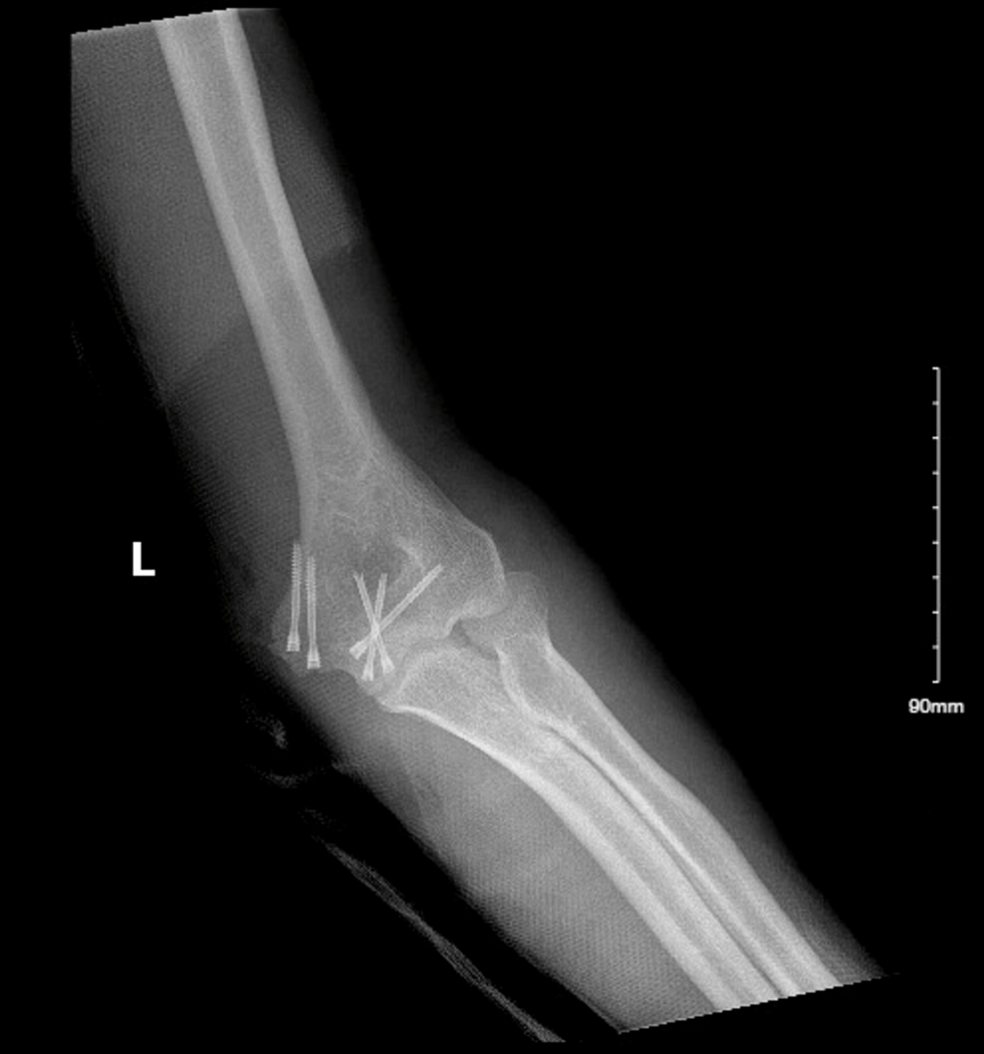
X-ray showing postoperative imaging of left humerus fracture repair
